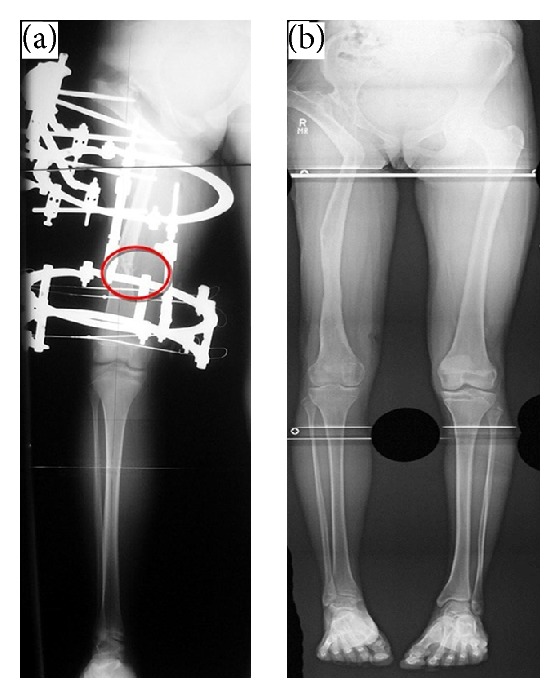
(a) Postoperative radiographs. (b) Postoperative radiograph after frame removal.
